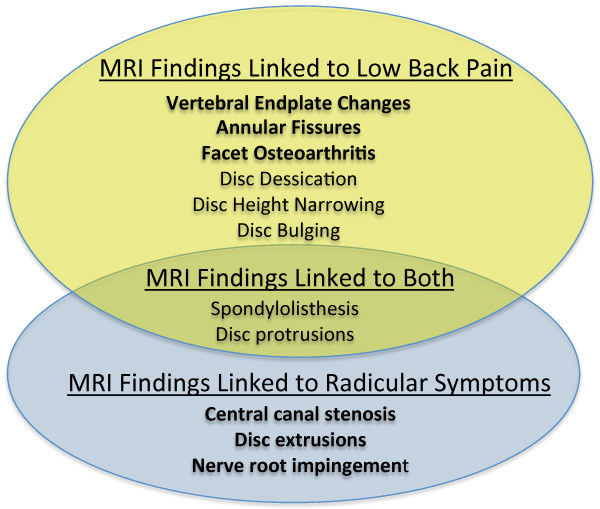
Conceptual links between MRI findings and spine-related symptoms*. *Primary MRI predictors of interest in bold.
